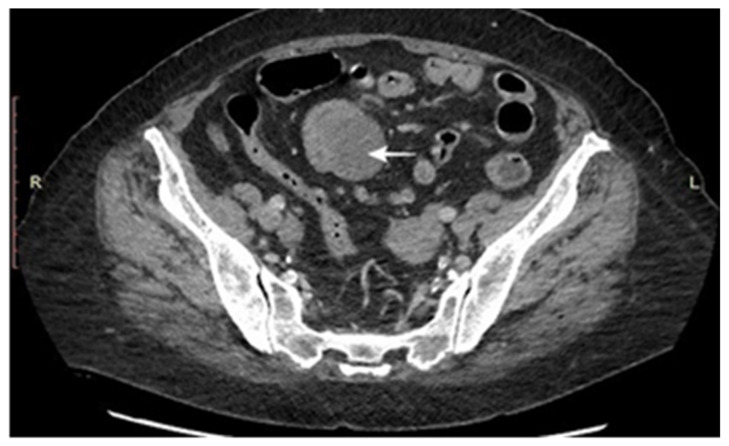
CT scan: tumoral mass with ileal location and predominant extraluminal extension, ileal luminal stenosis and upstream dilation of the small bowel, moderate enhancement, and mesenteric fat infiltration. Several mesenteric lymph nodes up to 1.4 cm.
